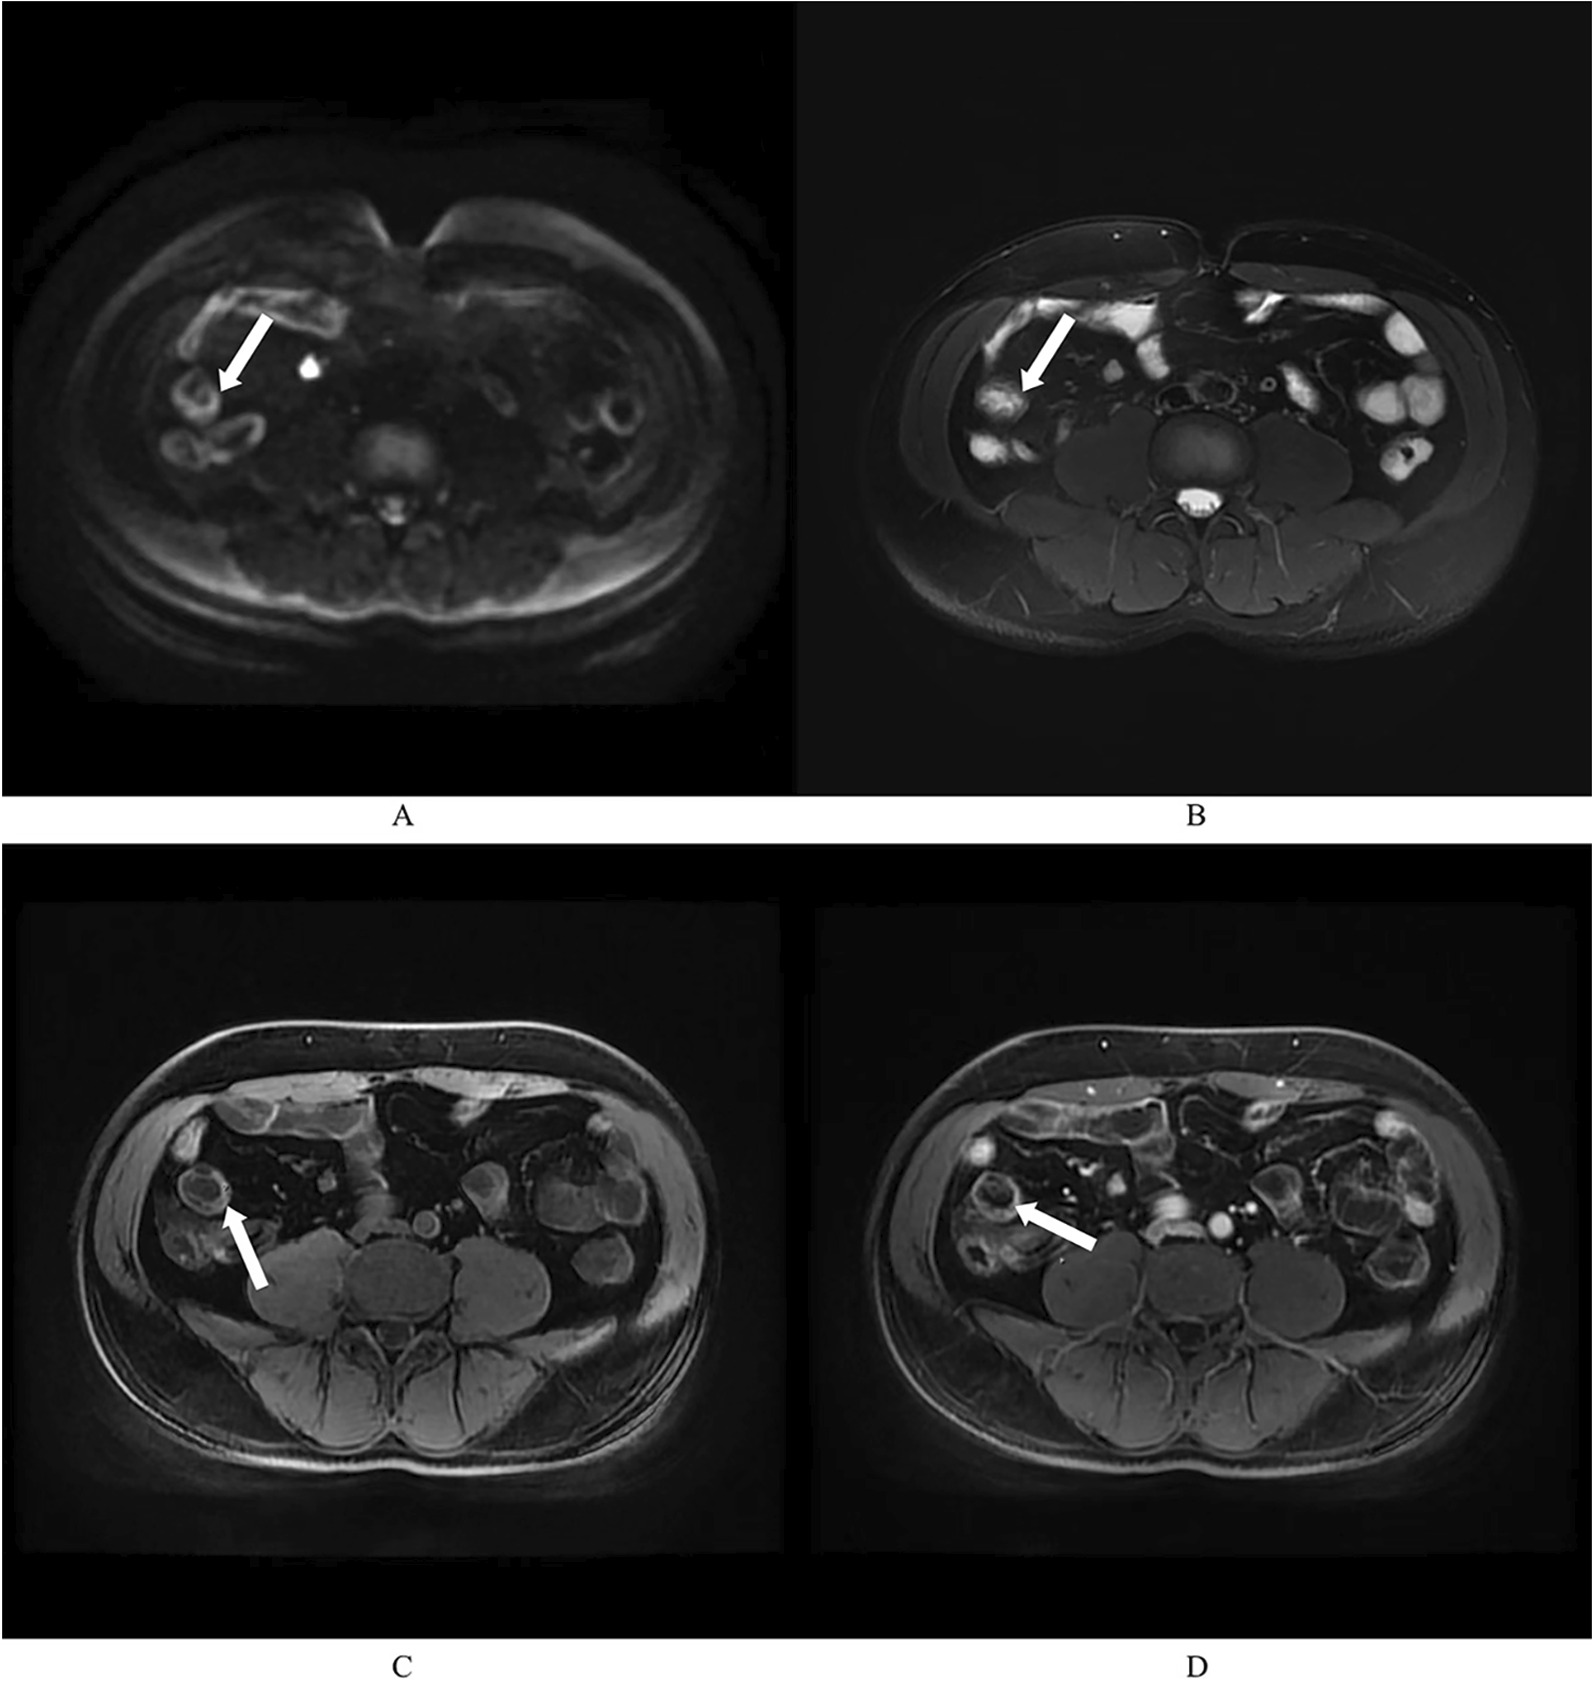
The presentation of MaRIA assessment by two radiologists. A 49-year-old male patient with Montreal classification of A3B1L1 was classified UG (tCDEIS = 8). A and B, axial DWI sequence (A) and axial T2-weighted single-shot fast spin-echo image with fat saturation (B) presented the abnormality of terminal ileum (arrow). C and D represent pre- and post-enhancement sequences, and the enhancement could be detected of the intestinal wall (arrow). The BWT measured by R1 (the senior) and R2 (the junior) was 4.75 mm, 4.00 mm, respectively, and the RCE assessed by R1 and R2 was 119.7, 93.6 separately. R1 considered that the edema could be detected, while R2 did not. No sign of ulcer was reported in both radiologists. MaRIA evaluated by R1 was 14.52, and 7.87 by R2. Obviously, R1 made an accurate assessment and R2 made an inappropriate diagnosis
